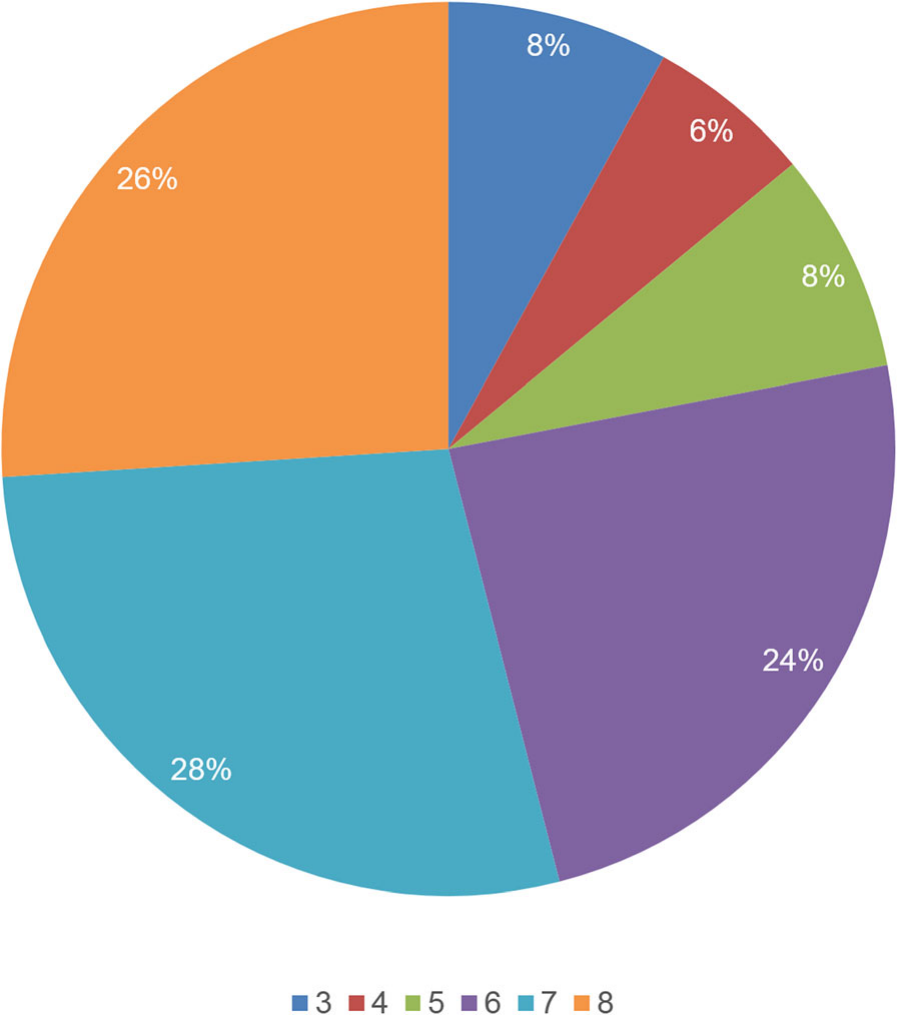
Number of foetal echocardiography views successfully obtained in each foetal heart with 5D Heart (*n* = 50). 3 to 8 means the total number of diagnostic planes retrieved from one foetus, and the success rates are shown in the pie chart
